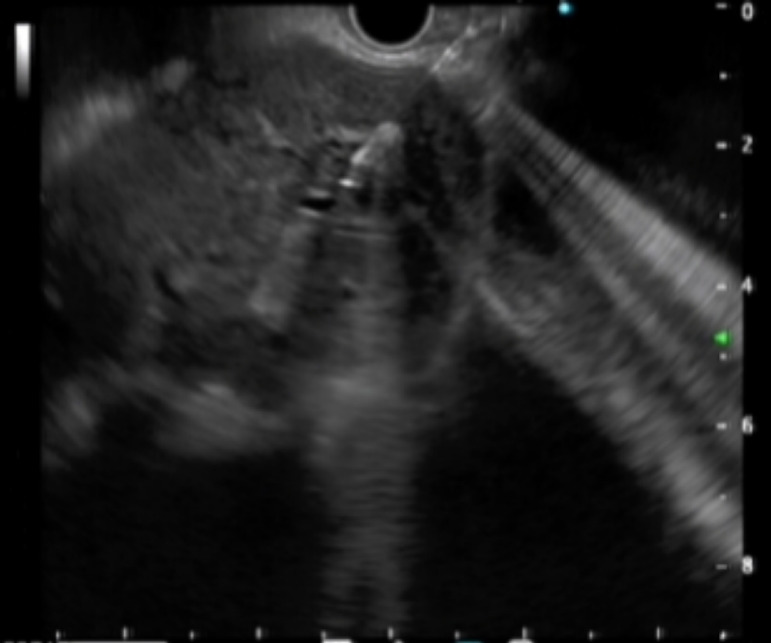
Ultrasound endoscopically guided intrahepatic bile duct puncture.
